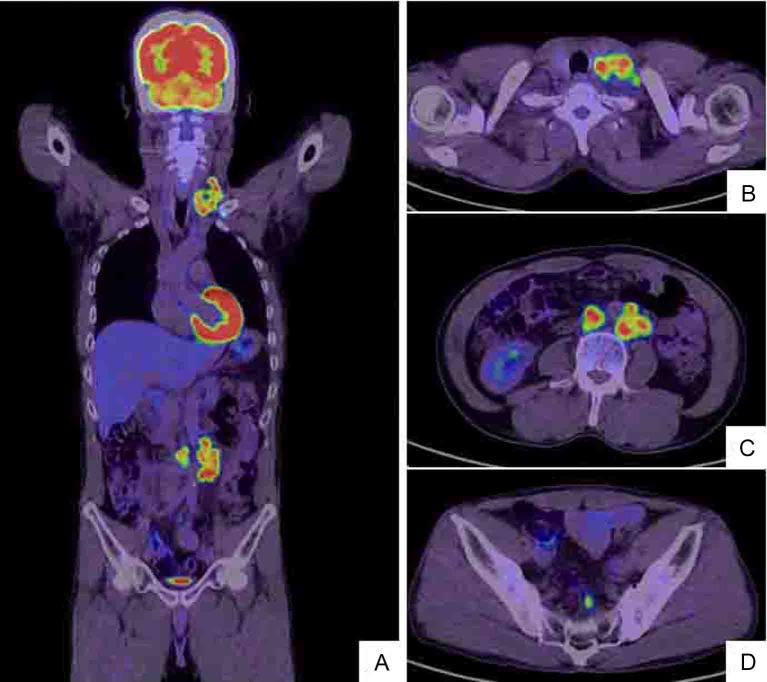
Whole body positron emission tomography (PET)/CT scan (A) shows hot lesions of multiple LN metastases to left cervix and left supraclavicular fossa (B) as well as enlarged left abdominal periaortic, inferior mesenteric and surrounding (C) and colic (D) LNs. The PET/CT and CT findings are identical (Fig. 1).
